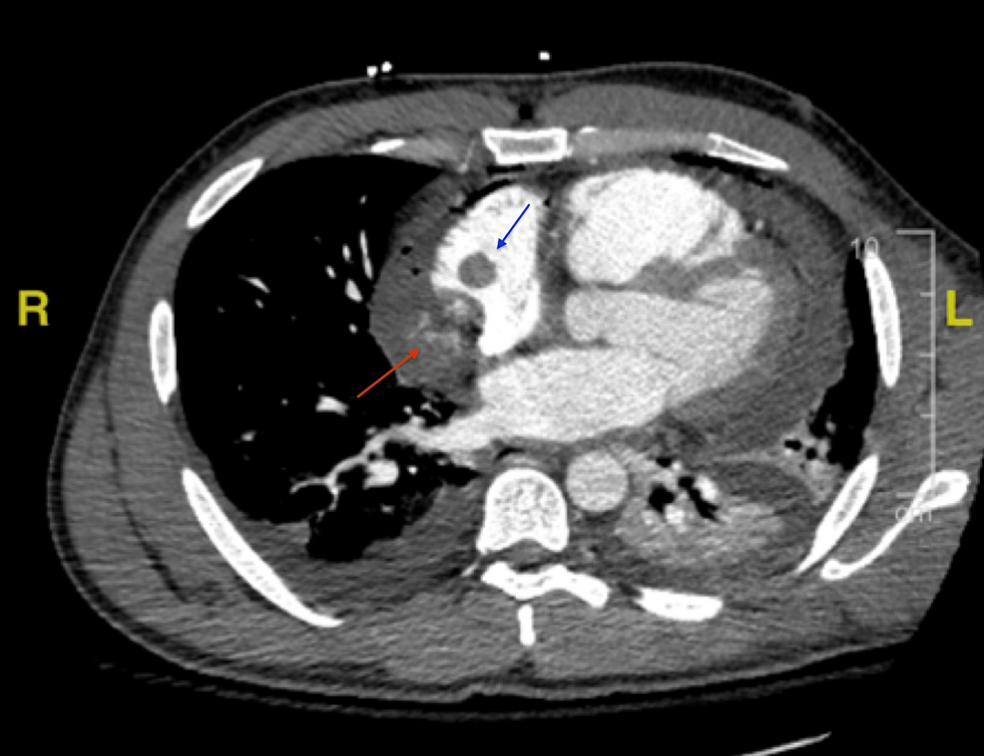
CTA chest depicting small amount of heterogeneous hyperdense material along the medial aspect of the right atrium (red arrow) and a 1.4 cm mass at the superior medial aspect of the right atrium (blue arrow). CTA, computed tomography angiogram
